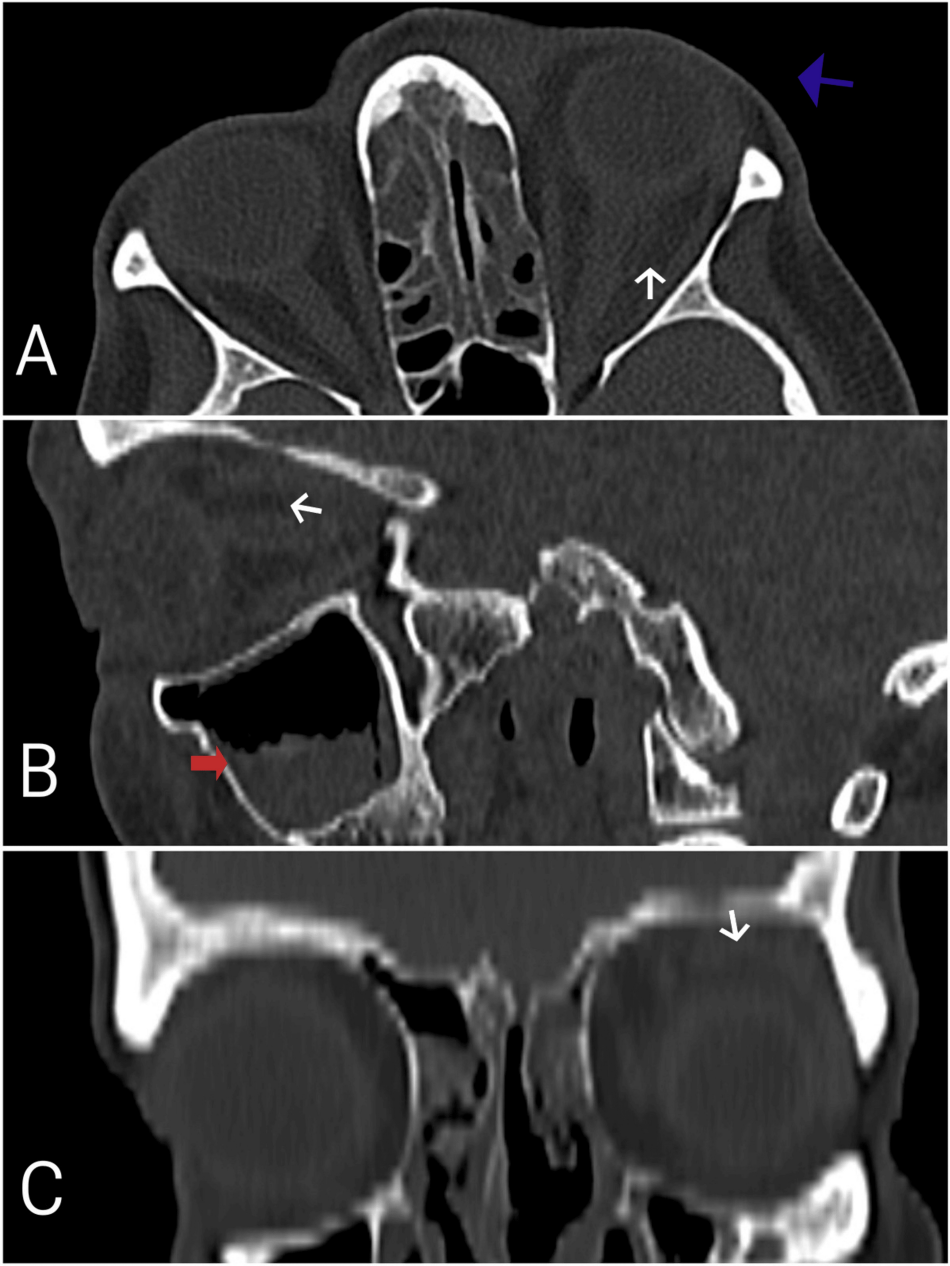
Axial (A), sagittal (B), and coronal (C) sections of a bilateral non-contrast orbital CT scan at the patient's initial hospital admission showing left orbital cellulitis (white arrows), proptosis of the left eye (blue arrow), and marked ethmoidal and maxillary sinusitis (red arrow).
